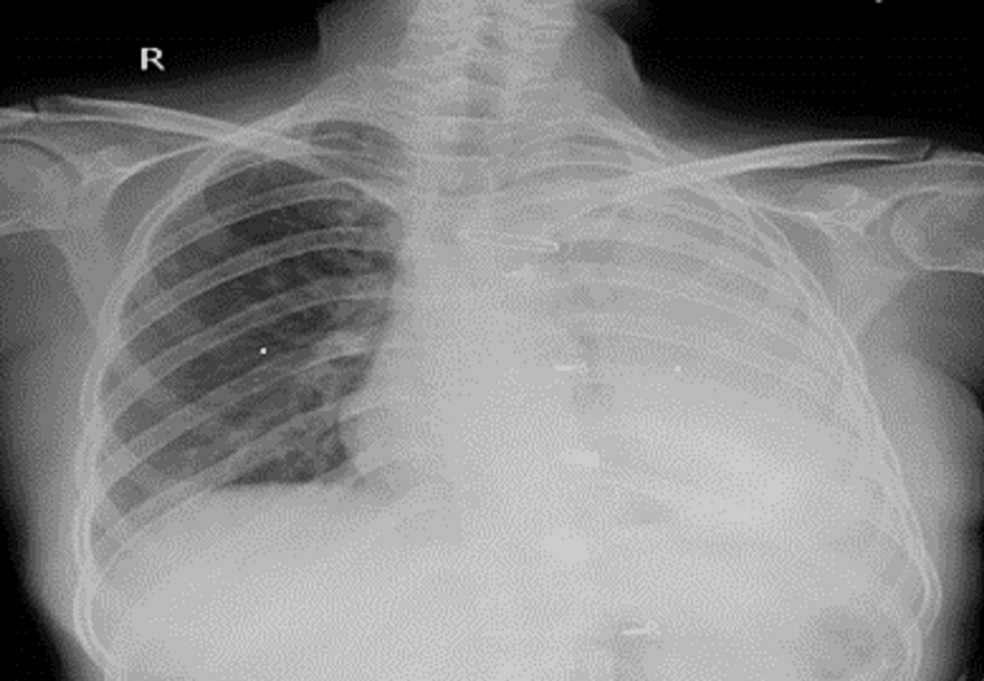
Chest X-ray showing left opaque hemithorax
